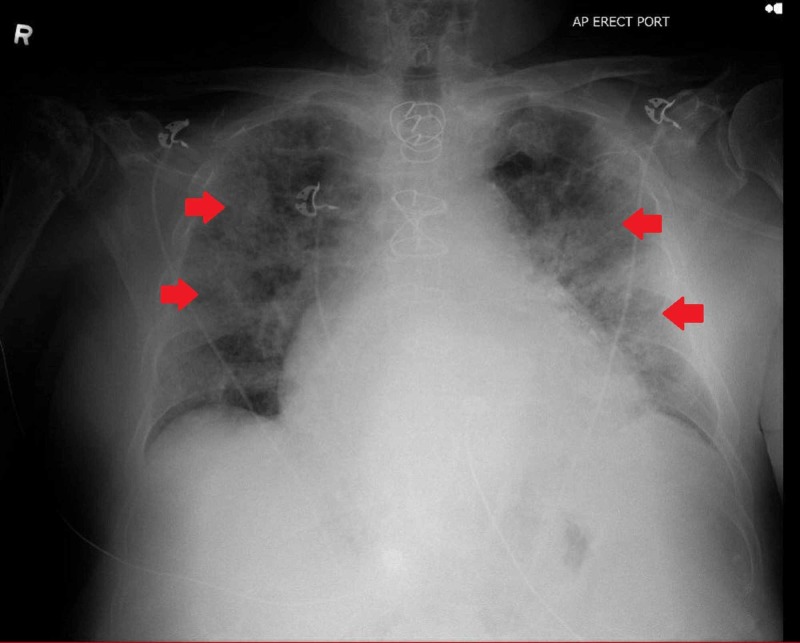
Chest X-ray on day 2 of admission revealing worsening infiltrates in bilateral lung zones
